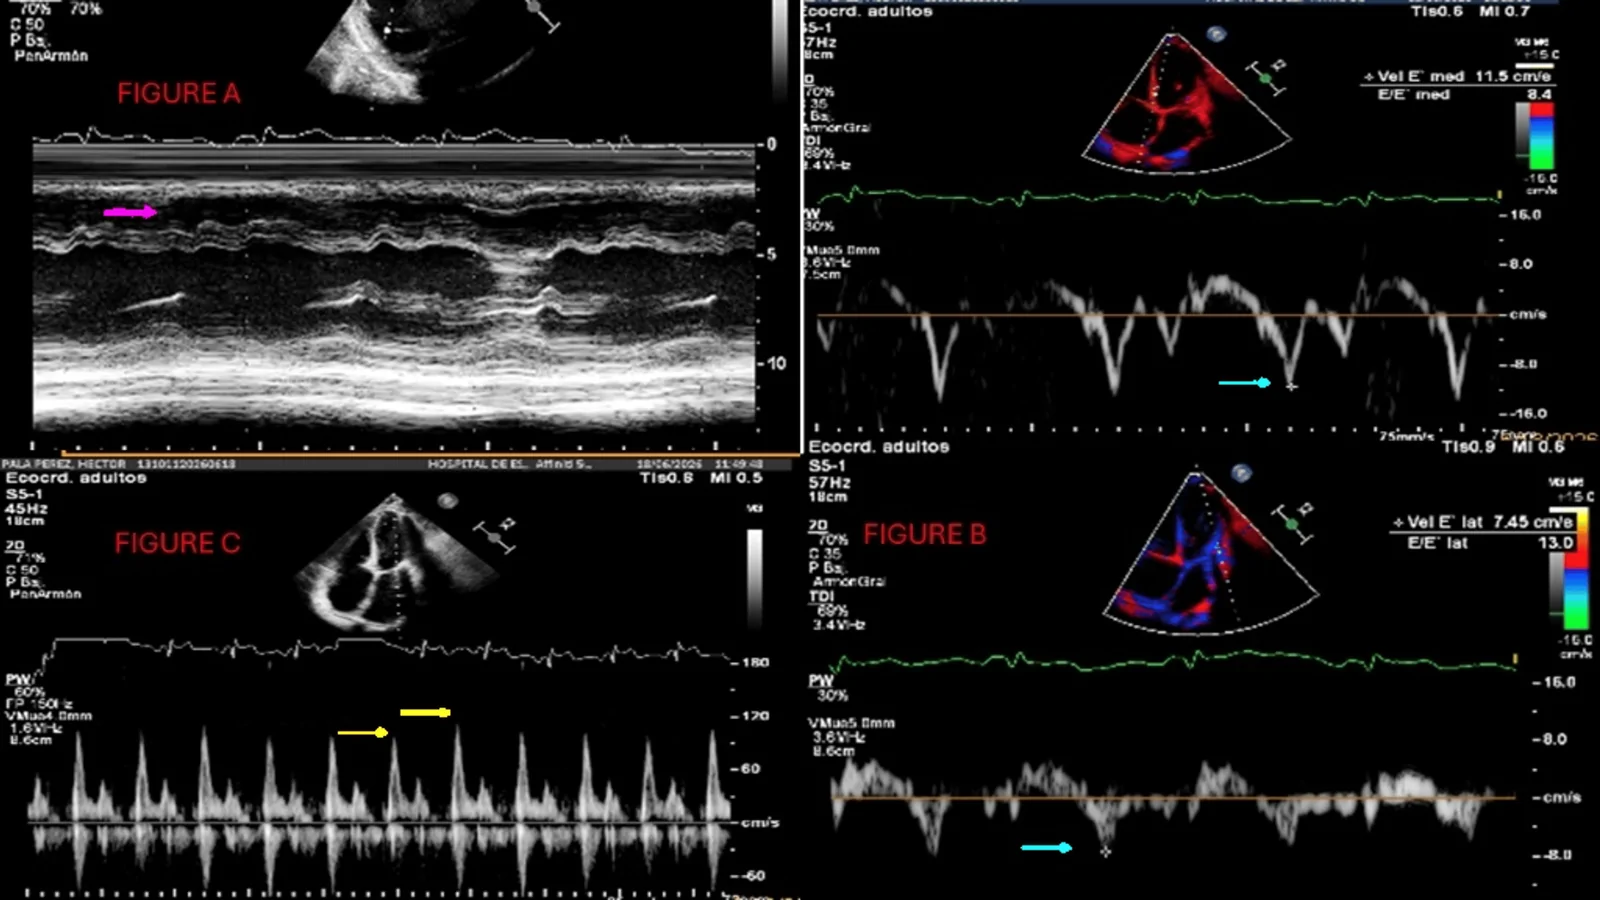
Pathognomonic functional echocardiographic and tissue Doppler findings (A) Echocardiogram (M-mode): The magenta arrow indicates the abrupt early diastolic septal bounce. (B) Tissue Doppler: The cyan arrows confirm annulus reversus (septal E' velocity paradoxically higher than lateral E'). (C) Transmitral Doppler: The yellow arrows show the 30% respiratory variation in E-wave velocity.
